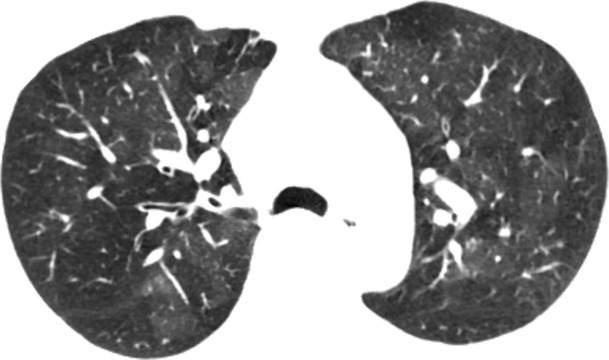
Bronchial obstruction due to asthma. Axial CT image in a patient during an asthma exacerbation demonstrating a mosaic attenuation pattern of the pulmonary parenchyma consistent with air-trapping
